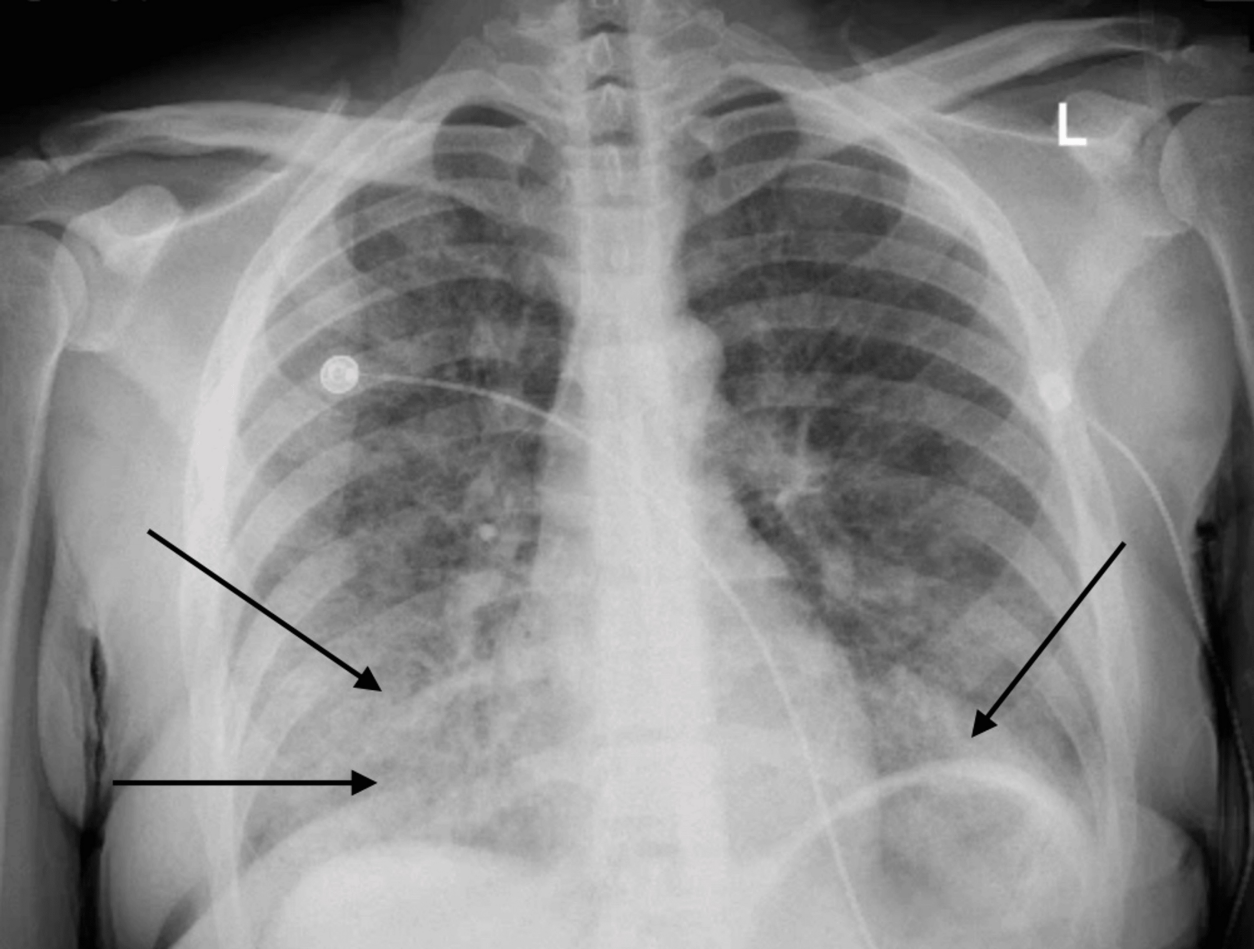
Initial chest X-ray. Black arrows showing bilateral lower lungs infiltrates worse on the right
